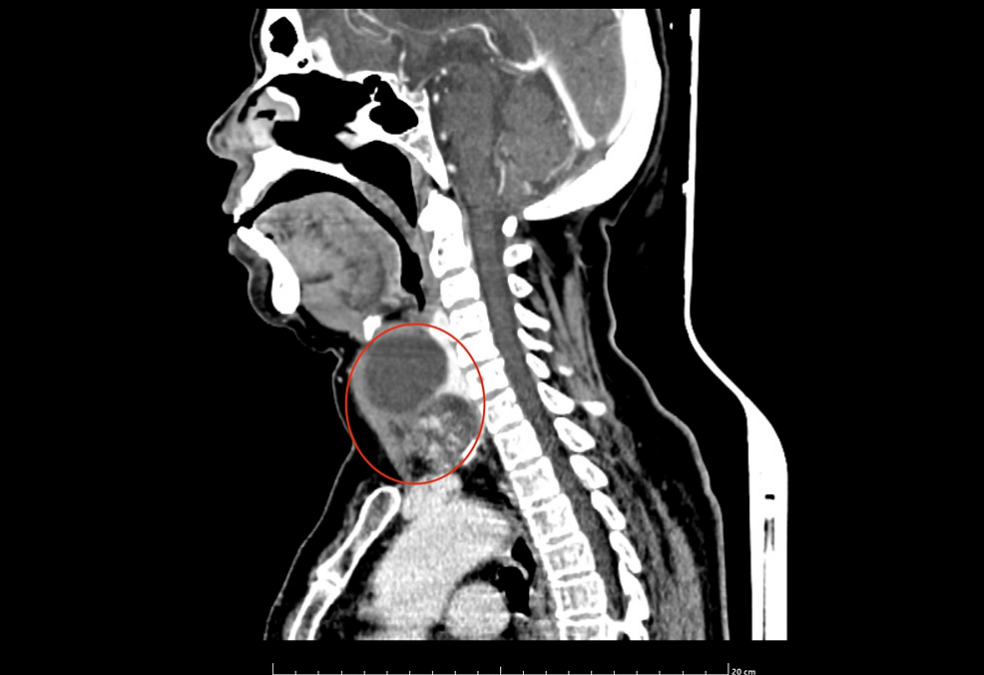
CECT of the neck showing lesion with a rim of calcification CECT: Contrast-enhanced computed tomography
